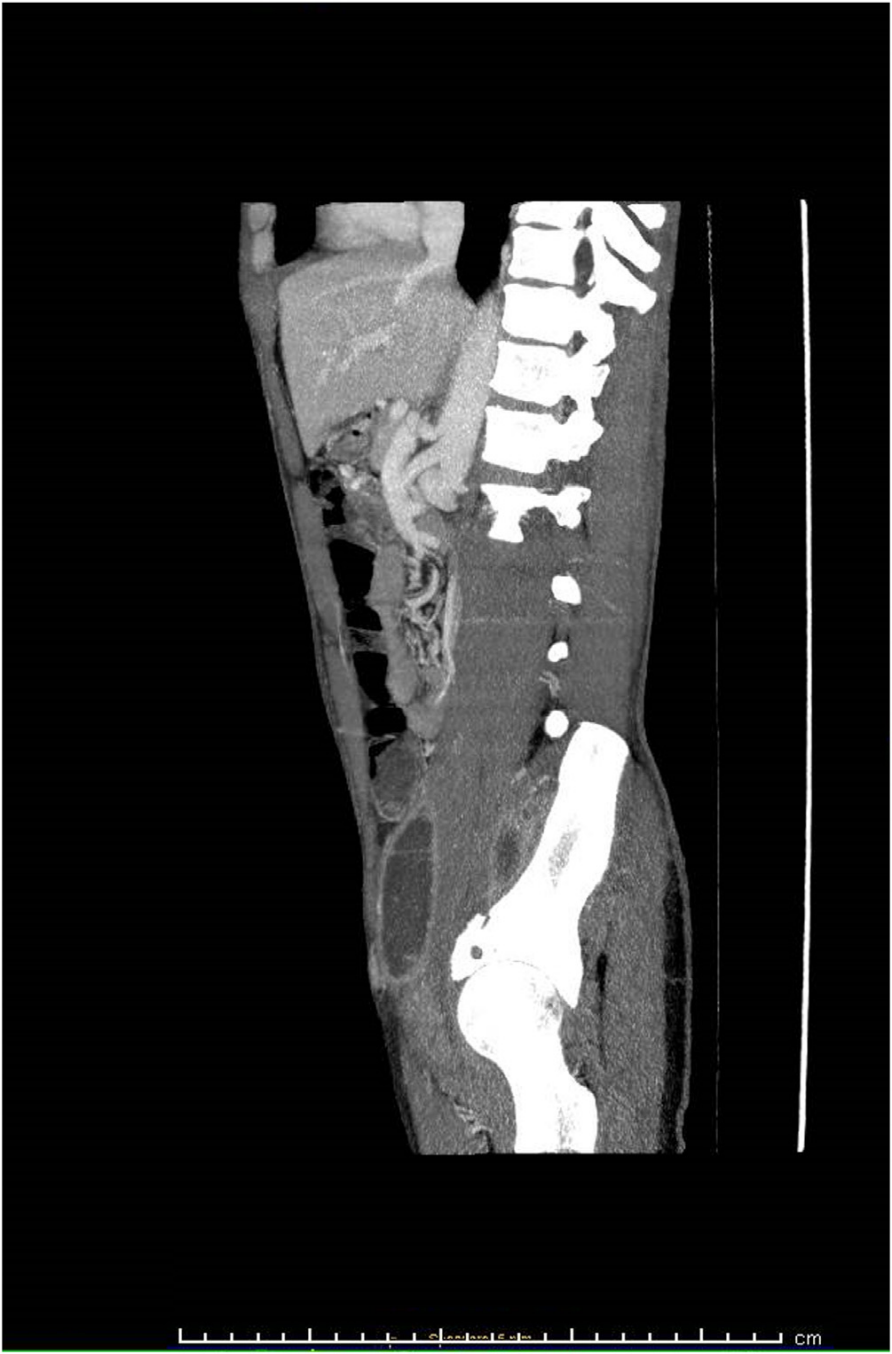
CT-scan sagittal view: the abscesses extended cranio-caudally up to 9 cm in length.
